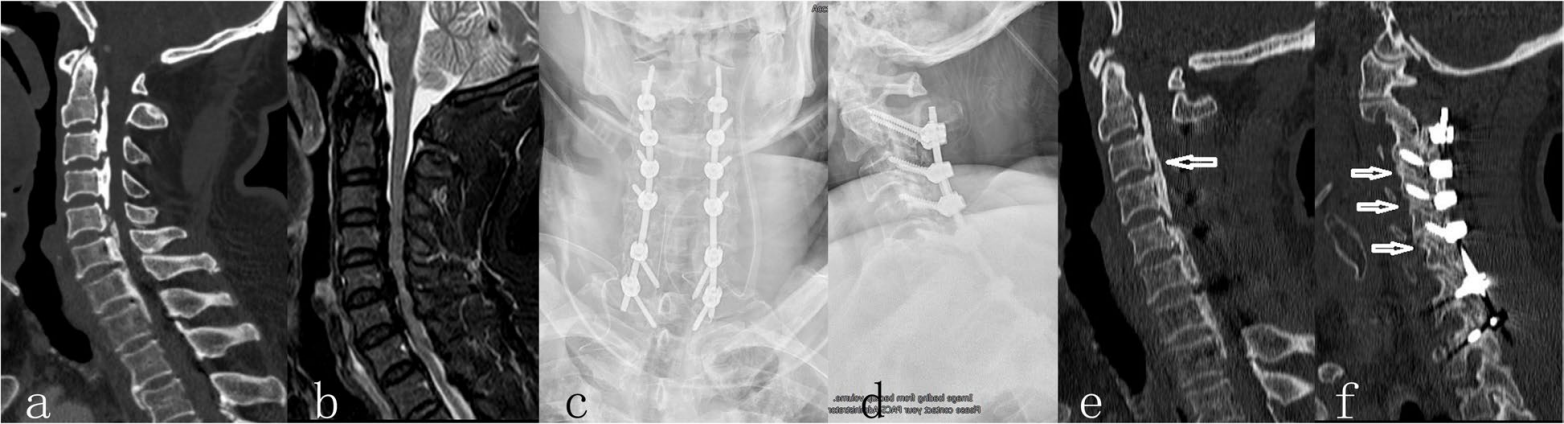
A 63-year-old male patient with multilevel, mixed-type ossification of the posterior longitudinal ligament. Preoperative computed tomography scan showed ossification of the posterior longitudinal ligament involving C2-T1 (a). Preoperative magnetic resonance image showed the pathological extent involving 6 intervertebral levels (b). He accepted crossing the cervicothoracic junction fusion from C2 to T1 and acquired sufficient decompression from C2 to C7 (c, d). Computed tomography scan of 12 month after surgery showed progression of ossification of the posterior longitudinal ligament (e). Computed tomography scan of 12 month after surgery showed fusion in the facet joints (f)
